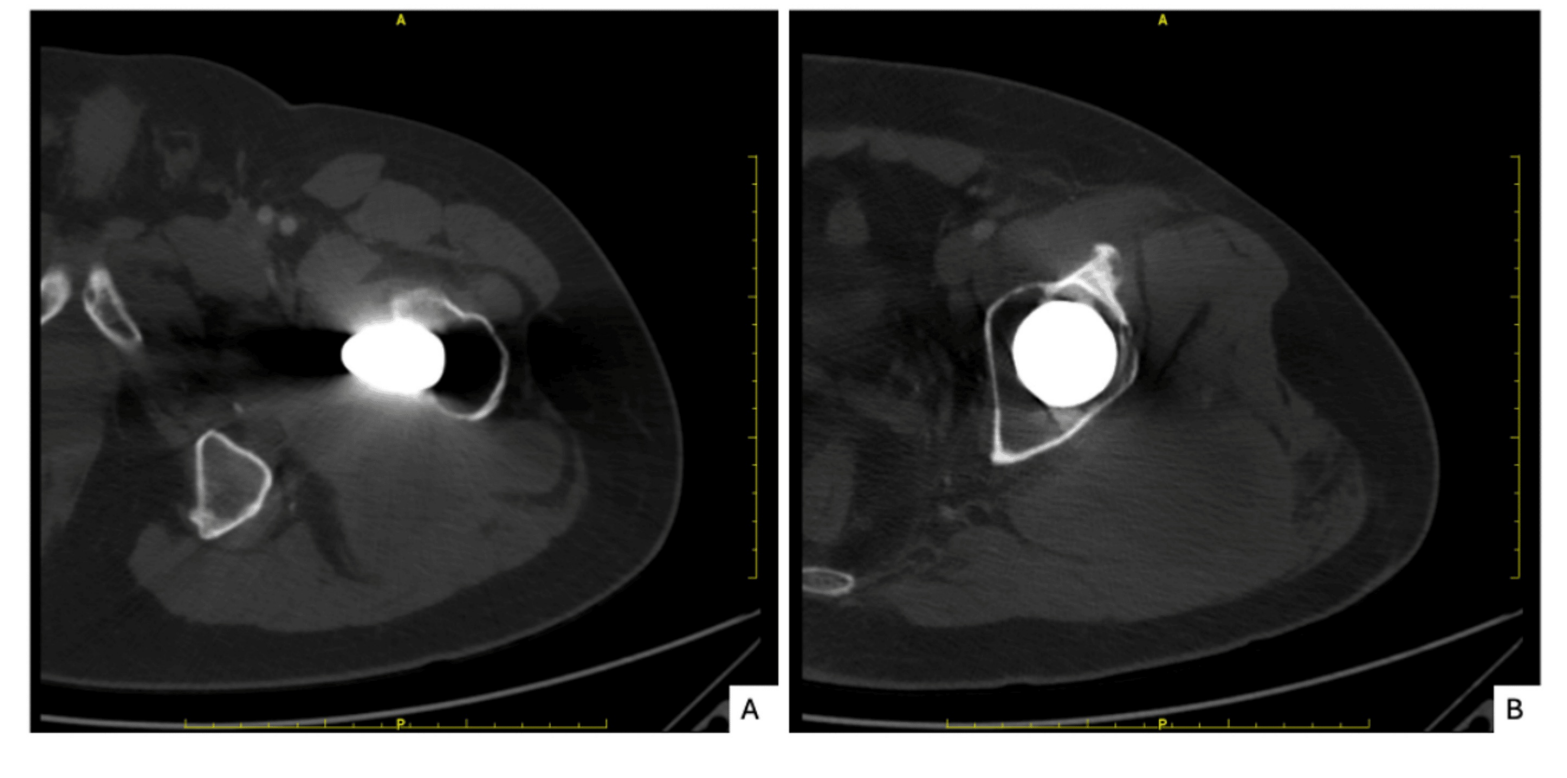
Axial contrast-enhanced CT images of the left hip (A) Axial CT image demonstrating periprosthetic lucency surrounding the femoral stem. (B) Axial CT image demonstrating posterior cortical hypertrophy and surrounding sclerosis involving the proximal femur in the trochanteric region.
